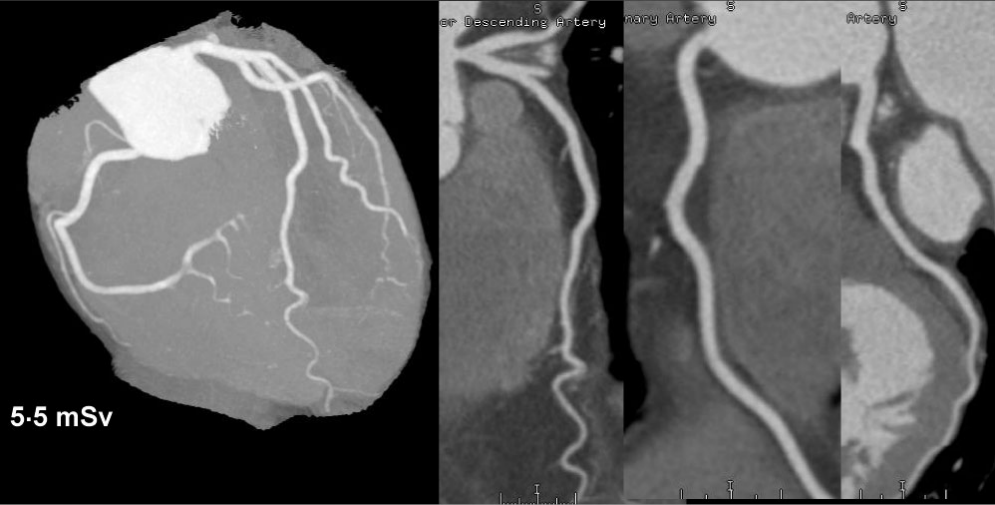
Example of sequential CT angiography with normal result. Radiation dose was 5.5 mSv.
